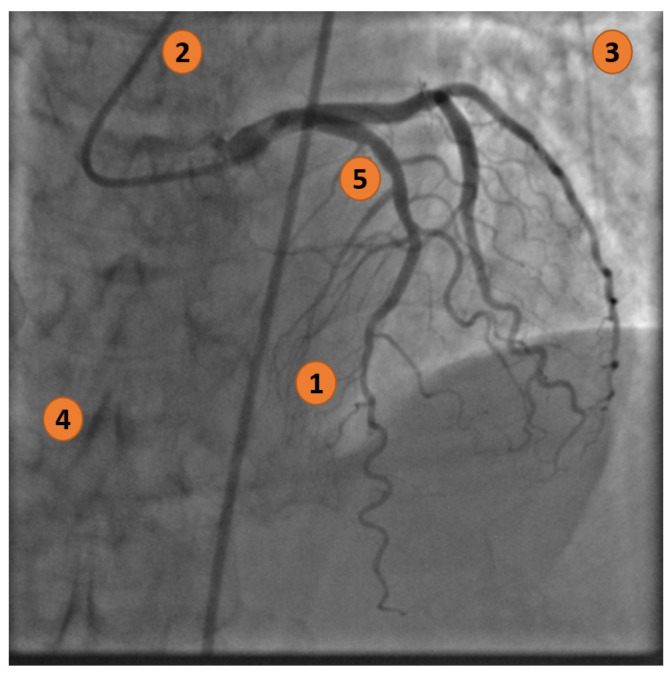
X-ray coronary artery with the following characteristics: (1) Zones with a low signal-to-noise ratio; (2) Interfering background structures (catheter); (3) Non-uniform illumination; (4) Artifacts and noise; and (5) Vessel junctions and bifurcations.
